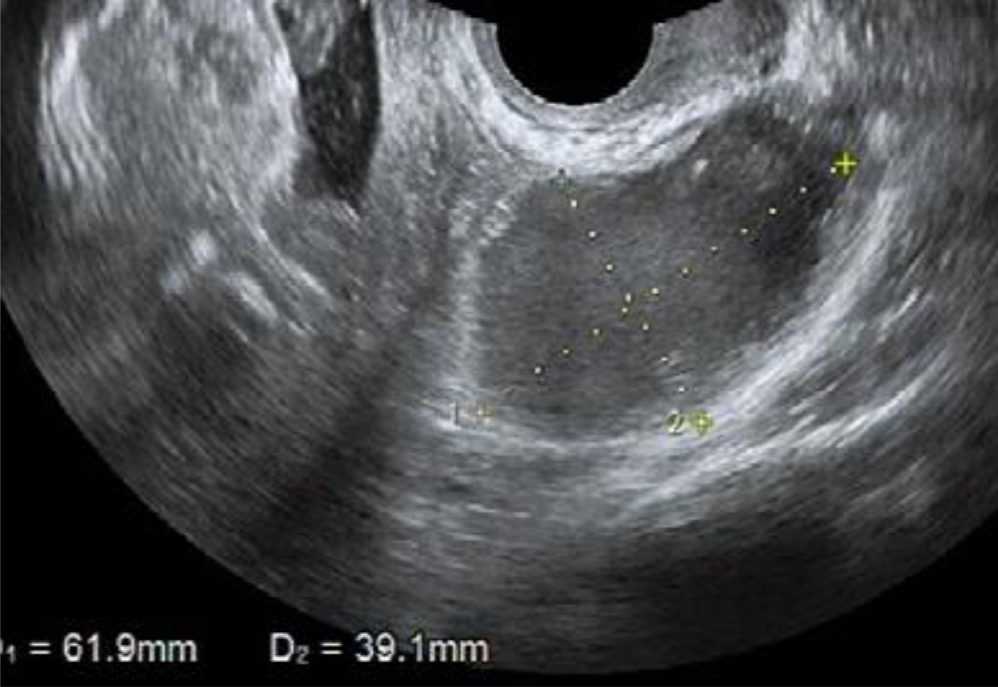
Ultrasound imaging at 16 weeks of pregnancy. The cyst increased in size to 60 mm.
